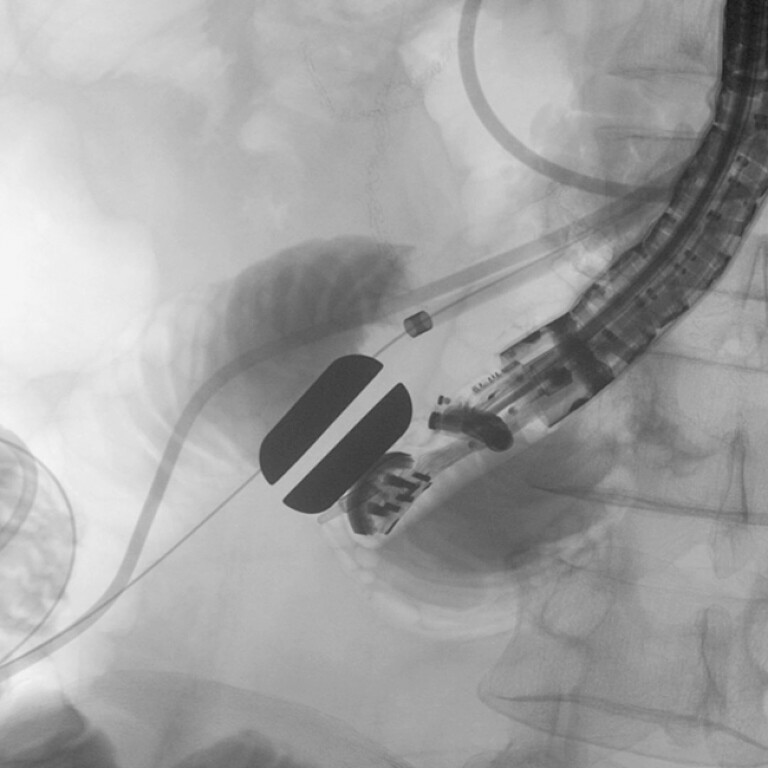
EUS-guided magnetic side-to-side entero-enterostomy was performed. EUS, endoscopic ultrasonography
